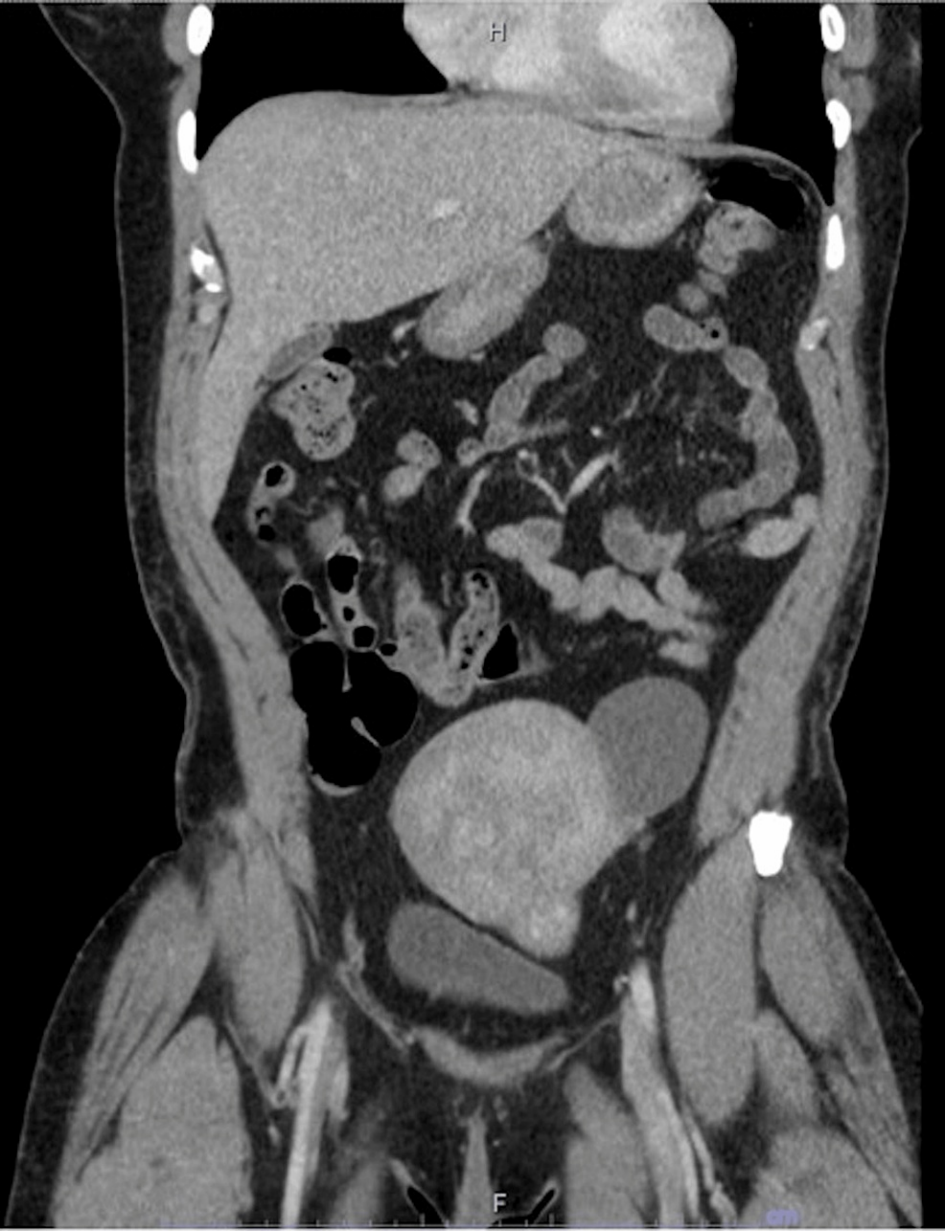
Coronal CT image of the abdomen and pelvis without any sign of tumor burden or metastatic disease (more posterior).
